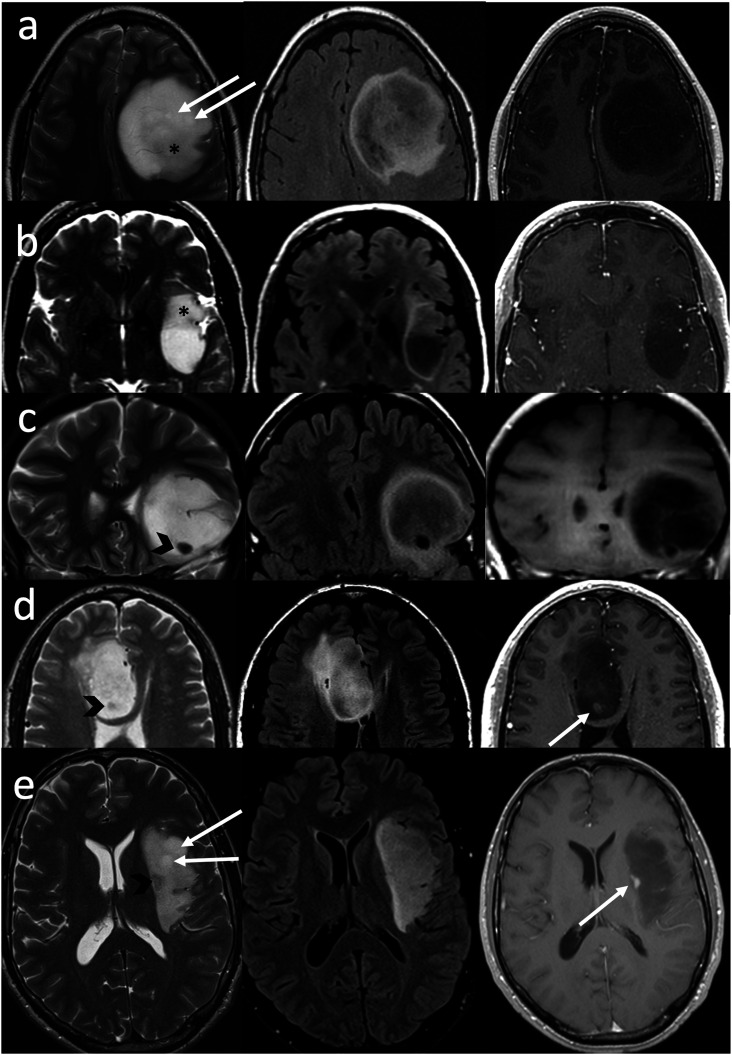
IDH-mutant, 1p/19q non-codeleted gliomas in a 26-year-old female (a), a 43-year-old female (b), a 36-year-old male (c), a 52-year-old male (d), and a 42-year-old male (e). The lesions do not show the “classic” T2FMM sign, as they meet the FLAIR criteria but not the T2WI criteria. On T2WI (left column), they lack “complete homogeneous hyperintensity” due to small macrocystic components (arrows, left column) or relatively hypointense regions/foci (asterisks and arrowheads, left column). All lesions meet the FLAIR criteria, as they demonstrate relatively hypointense signal except for a thin peripheral rim (middle column). As mentioned, homogenous signal is not a requirement for the FLAIR sequence like it is for the T2WI. This pattern (heterogeneous T2, hyperintense FLAIR rim) has been described as the “geographic T2FMM sign” by Throckmorton and colleagues.^
